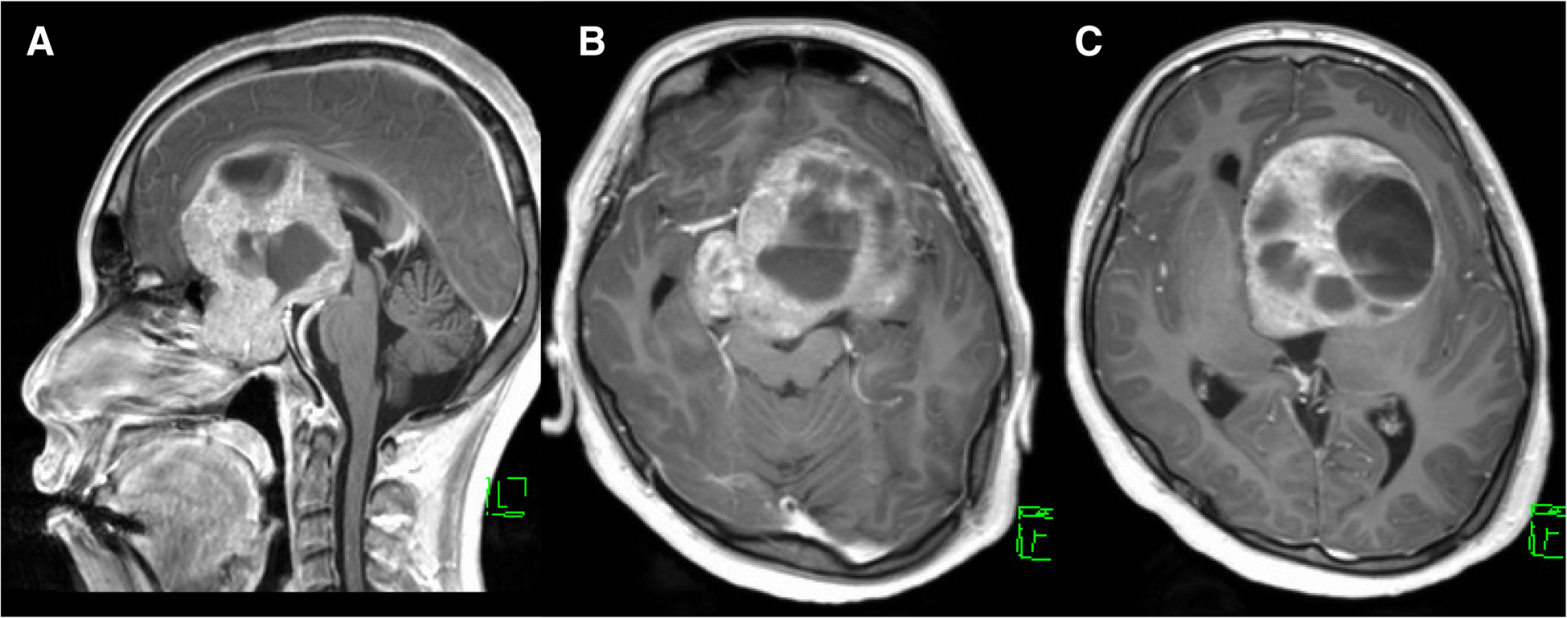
Gadolinium-enhanced T1-weighted images before the initiation of dopamine agonist therapy. a Sagittal view. b and c Axial images. Magnetic resonance imaging showed a tumor that arose from the pituitary and extended bilaterally through the anterior skull base, the clivus, and the cavernous sinus
